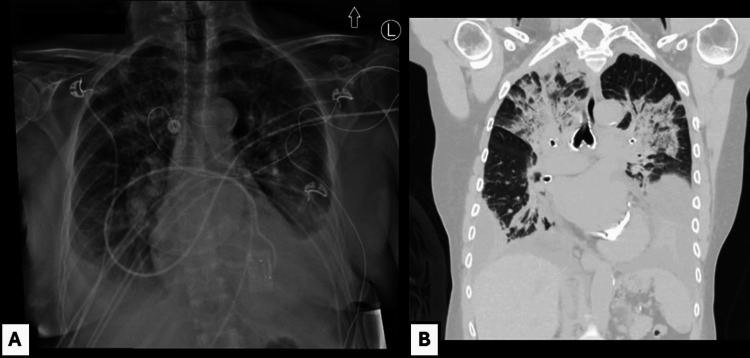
Thoracic radiology. (A) Chest X-Ray, anteroposterior, performed nine days after admission demonstrating bilateral pleural effusions. (B) Computed tomography (CT) of the chest (lung window) performed 11 days after admission showing bilateral upper lobe consolidation.
